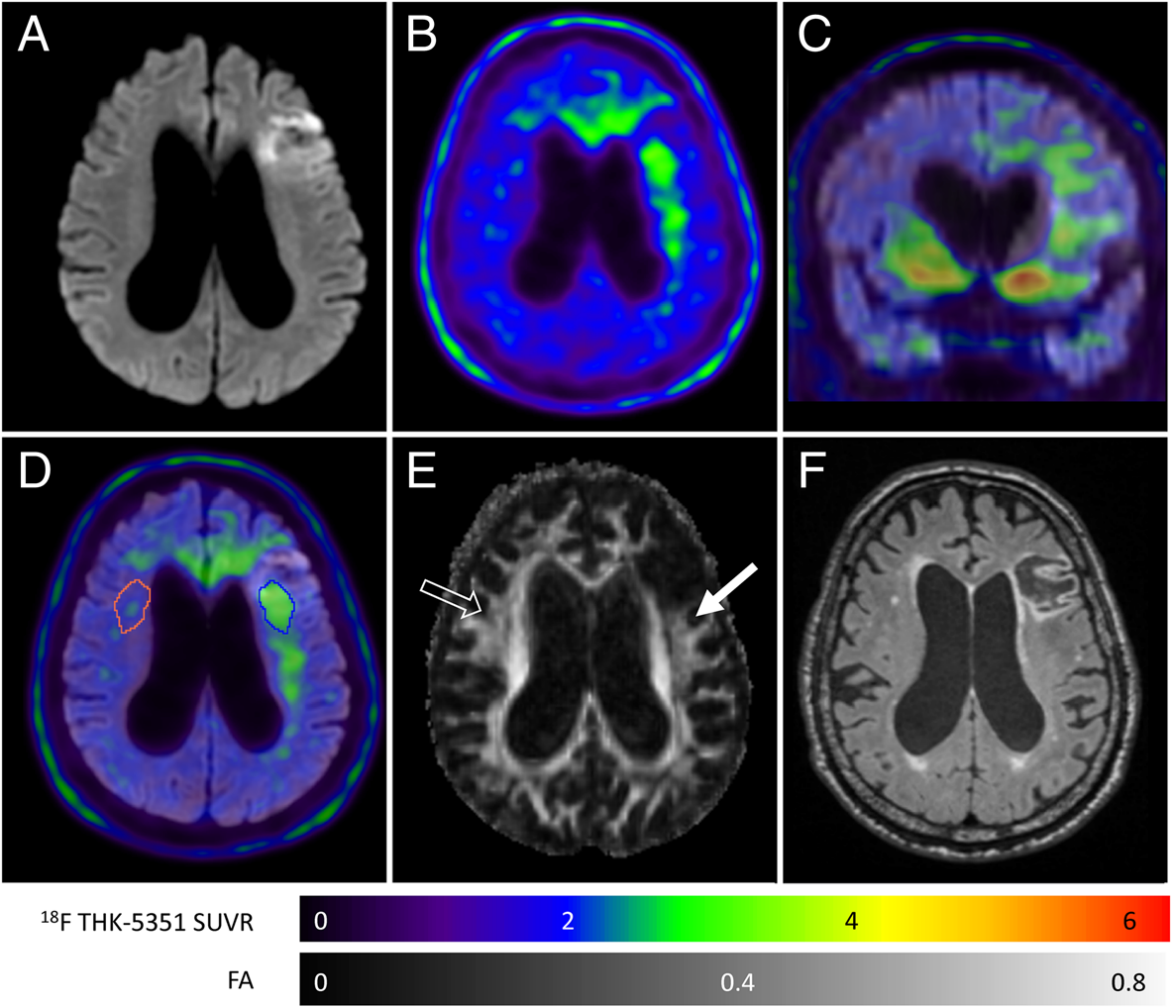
Multi-modality imaging characteristics in the patient (case 5) with a left frontal cortical infarction on diffusion-weighted imaging (DWI) (a). Low ^18^F-THK-5351 uptake is noted in the ischemic core, and high uptake is noted in the peri-ischemic area over the corona radiata, the genu of the corpus callosum, and in the bilateral frontal areas (b, c). The ROI for ^18^F-THK-5351 retention is drawn in the peri-ischemic area, and a mirrored ROI is placed in the contralateral hemisphere as the control (d). There is a decreased fractional anisotropy (FA) on diffusion tensor imaging (e) (filled arrow) in the peri-ischemic area with increased ^18^F-THK-5351 retention as compared to the contralateral side (empty arrow). No corresponding white matter change is noted on the follow-up FLAIR image (f)
